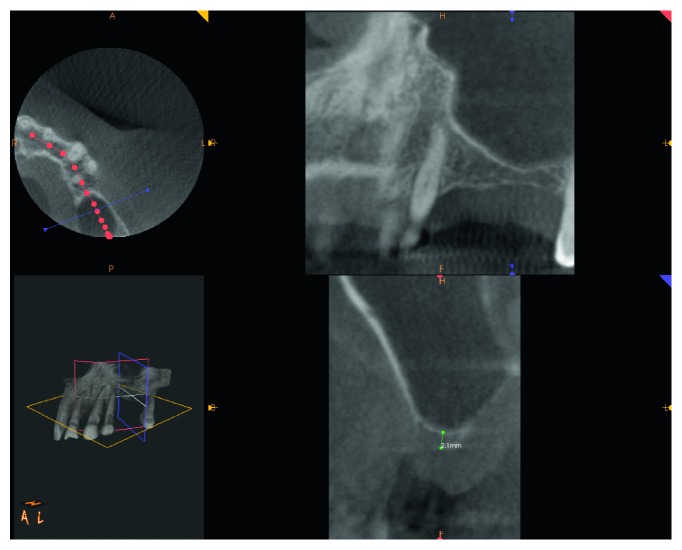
CT scan pre-op showing 2 mm of Bbh at distal site.
